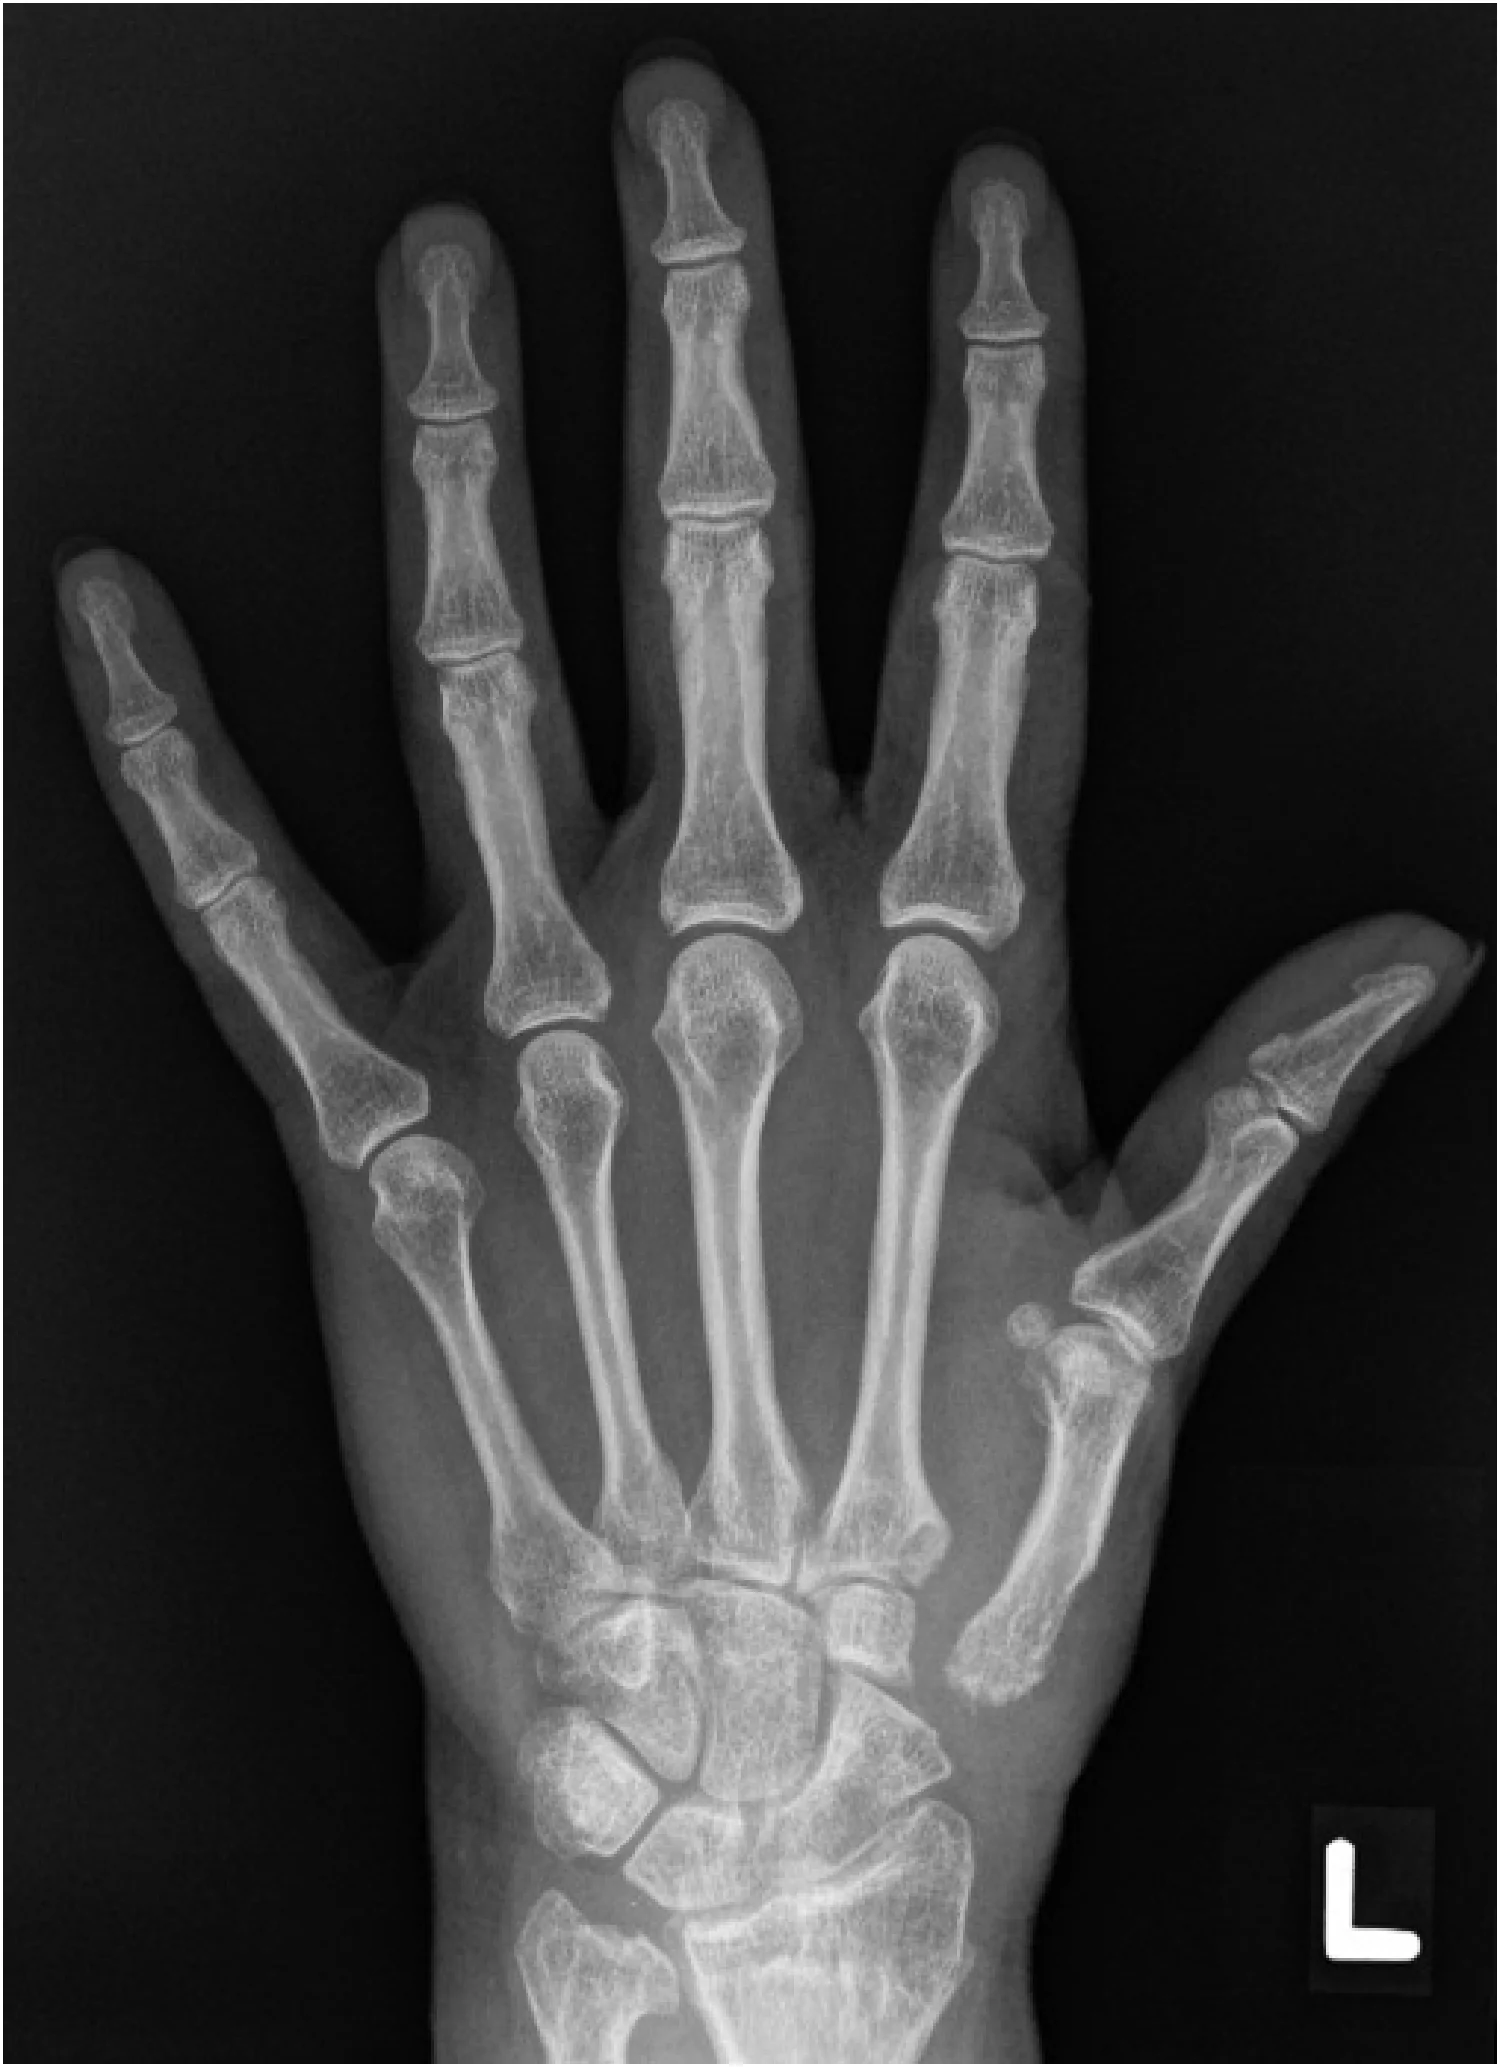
Anteroposterior radiograph of the left hand at 12 months following revision trapeziectomy and AlloDerm interposition arthroplasty. Proximal migration of the first metacarpal is present, which has remained stable compared to the immediate postoperative period and has not correlated with functional deterioration. The trapezoid is intact. The interposition space is maintained with no evidence of metacarpal base impingement.
